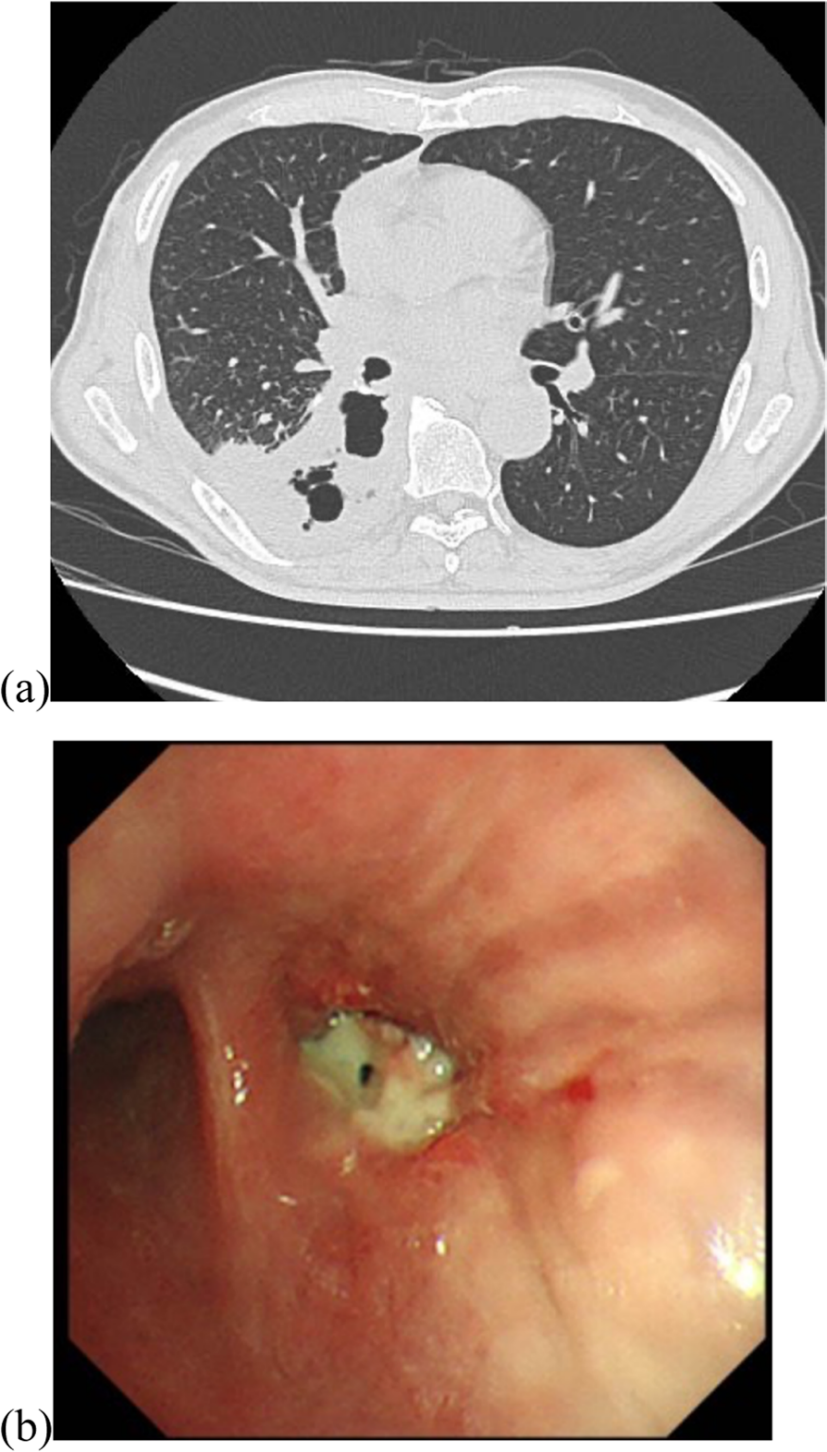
CT scan of the chest (a) and bronchoscopy (b) showing the fistula between the bronchial stump and the pleural cavity. CT, computed tomography
